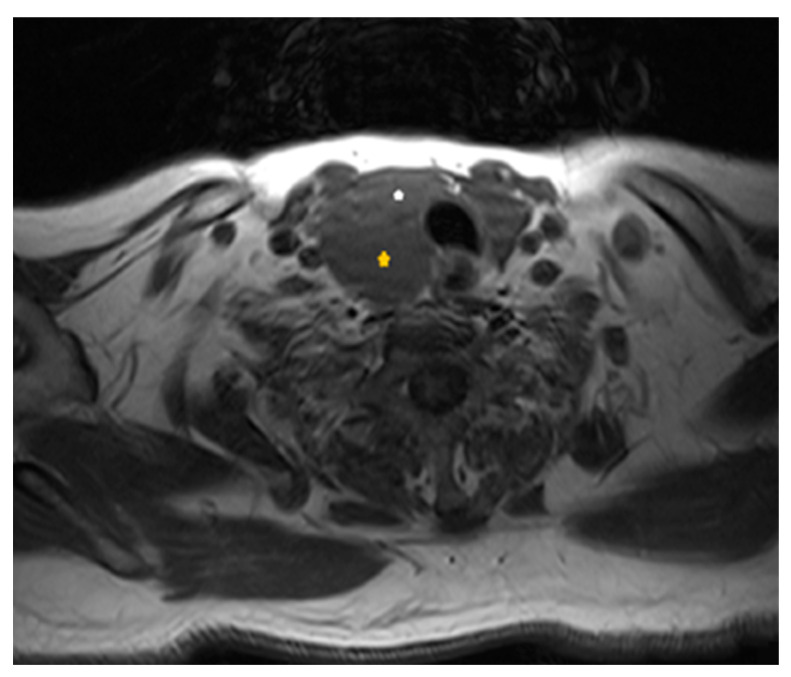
Axial T1-weighted scan at the level of the thyroid gland: yellow asterisk shows the extrathyroidal large parathyroid cystic mass, posteriorly to the right side of the thyroid gland (white asterisk).
